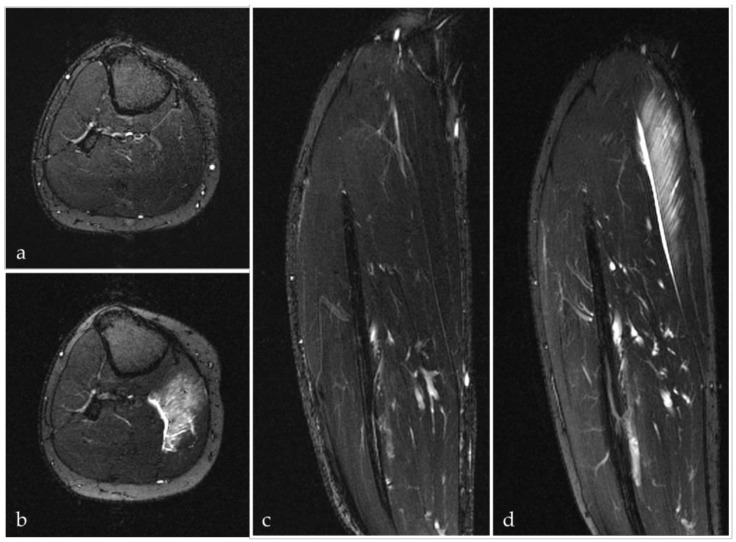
Axial (a,b) and coronal (c,d) T2-weighted fat-suppressed MRI images of the lower leg before (a,c) and 60 h after eccentric exercise (b,d) in the same participant. The increased signal intensity (b,d) reflects a rising fluid content in the gastrocnemius medialis muscle as equivalent of DOMS.
